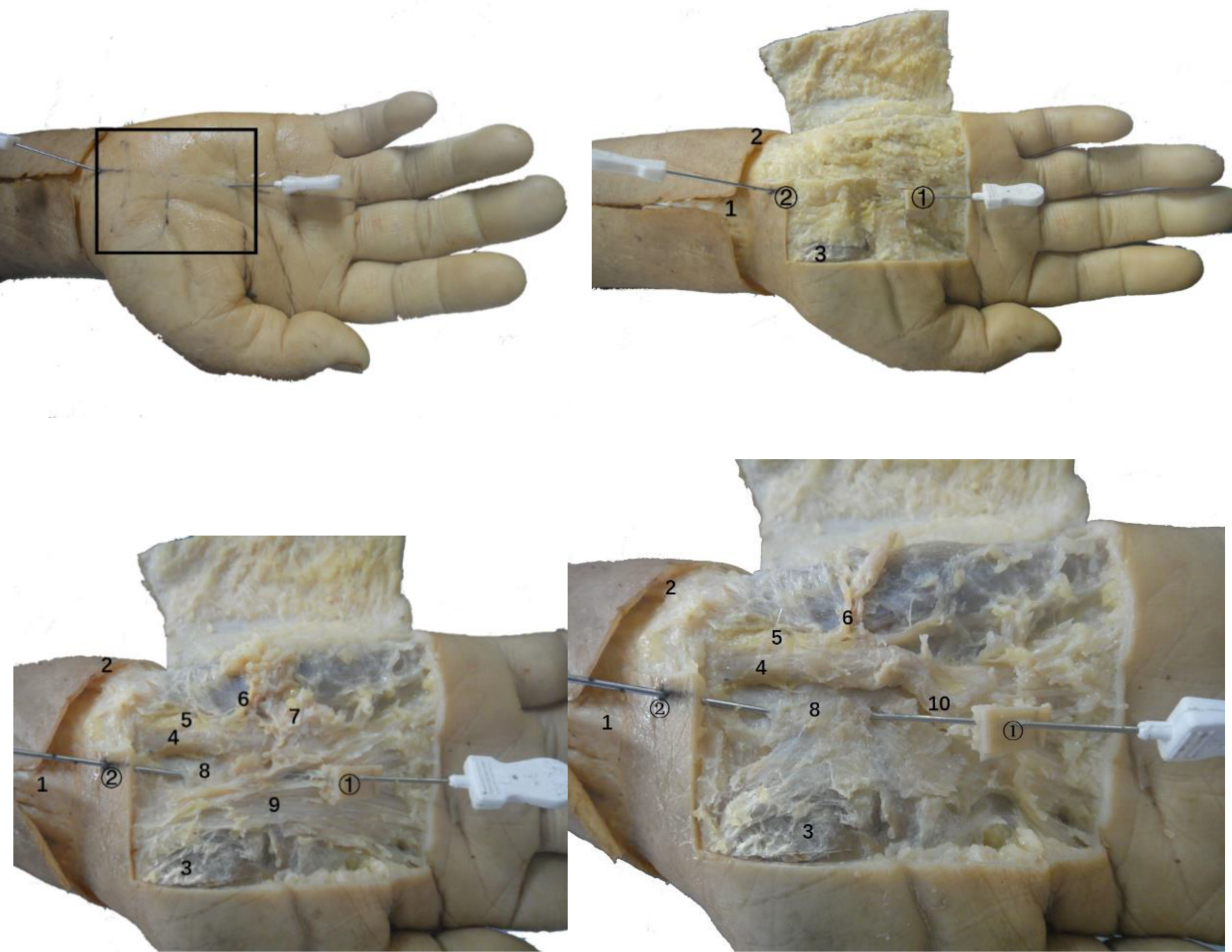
Anatomical view of the needle-knife release technique guided by ultrasound. 1. Palmar longus tendon; 2. Proximal transverse carpal striae; 3. Abductor pollicis brevis; 4. Ulnar artery; 5. Ulnar nerve; 6. Superficial branch of ulnar nerve; 7. Palmaris brevis; 8. Transverse carpal ligament; 9. Palmar aponeurosis; 10. Superficial palmar arch; ①, needle insertion point 1; ②, needle insertion point 2.
